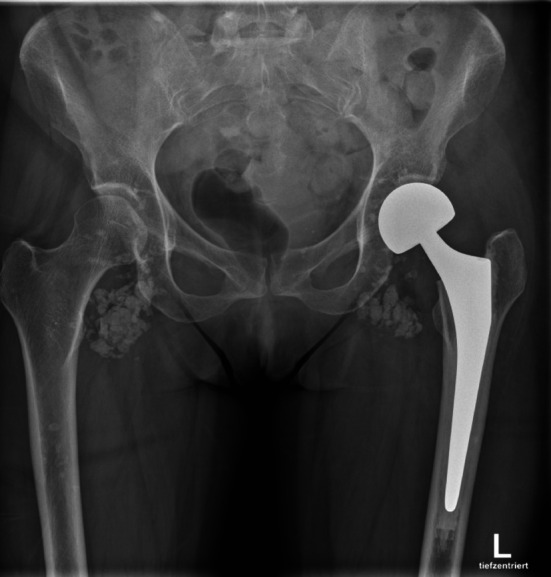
Anteroposterior pelvic radiograph demonstrating multiple bilateral clustered calcified subcutaneous lesions within the gluteal regions adjacent to the ischiopubic rami. The calcifications were identified incidentally during post‐operative imaging following left hip arthroplasty.
